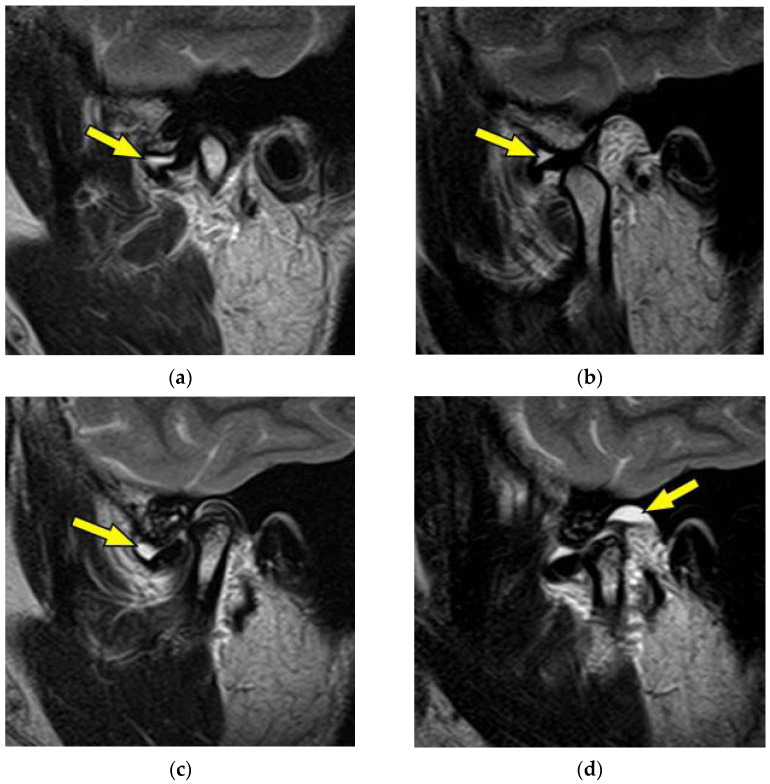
MR image of a 38-year-old woman with TMDs of right and left disc displacement without reduction. (a) Right TMJ: T2-weighted sagittal oblique cross-section imaging in the closed-mouth position; (b) right TMJ: T2-weighted sagittal oblique cross-section imaging in the opened-mouth position; (c) left TMJ: T2-weighted sagittal oblique cross-section imaging in the closed-mouth position; (d) left TMJ: T2-weighted sagittal oblique cross-section imaging in the opened-mouth position. Arrows indicate the region of joint effusion.
